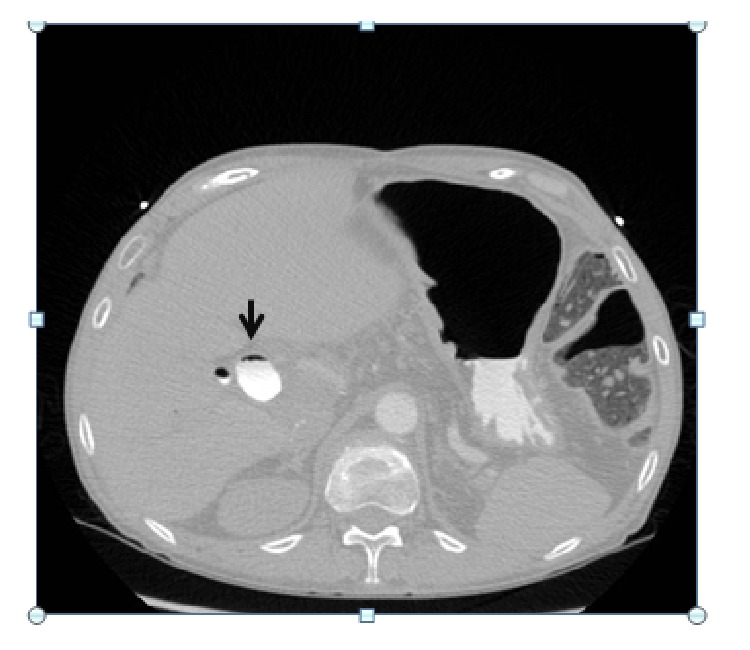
Contrast enhanced axial CT view showing air embolism (black arrow) inside intrahepatic portion of vena cava.
